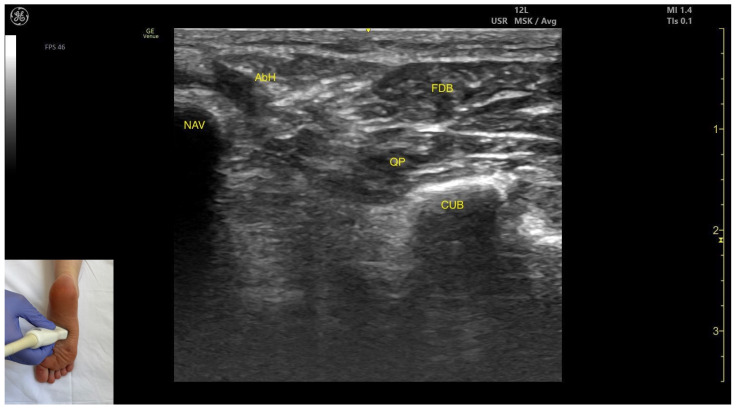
Ultrasound anatomy of the flexor digitorum brevis: NAV—navicular; AbH—abductor hallucis; FDB—flexor digitorum brevis; QP—quadratus plantae; CUB—cuboid.
